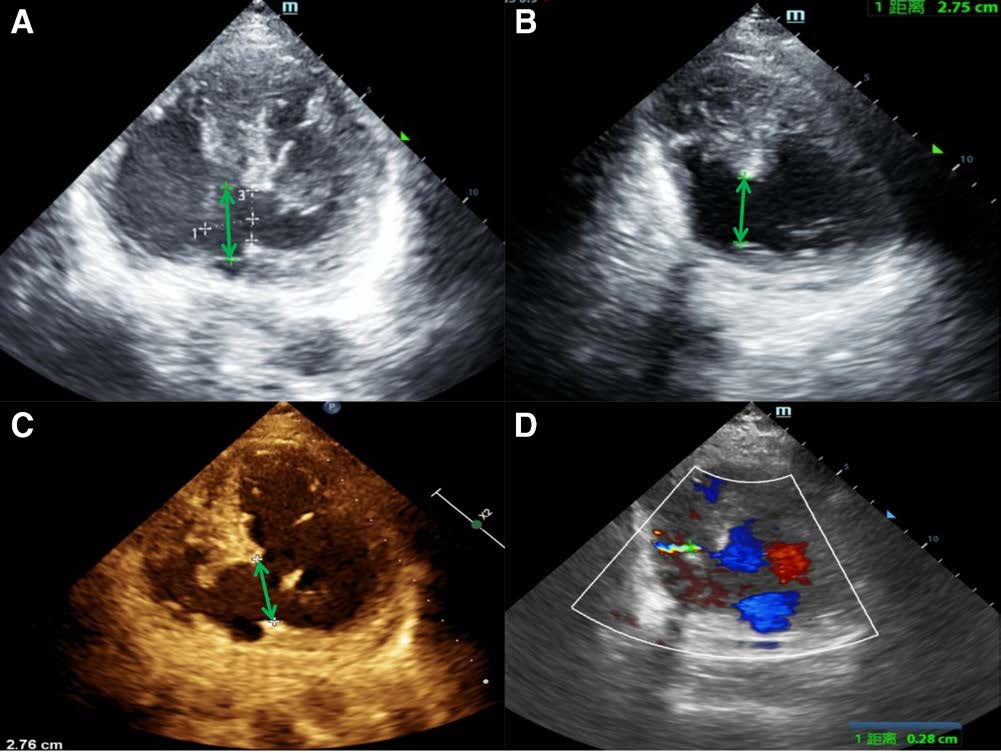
Ultrasound images on (A) day 0: a 17.1-mm defect in the ventricular septum; (B) day 10: the ventricular septal defect widened to approximately 27.5 mm at the left ventricular aspect; (C) day 17: the defect measured approximately 27.6 mm at the left ventricular aspect; and (D) day 27: postoperative image showing a residual defect at the inferior margin of the patch, measuring approximately 2.8 mm at the right ventricular aspect.
